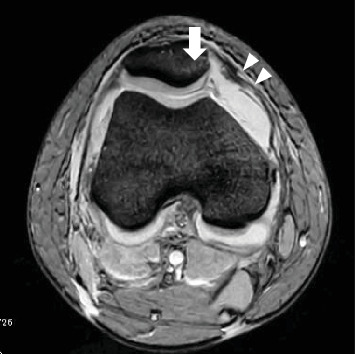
MRI images of the medial patellofemoral ligament (MPFL) injury (arrowheads) and slight bone bruises on the patella (arrow).
